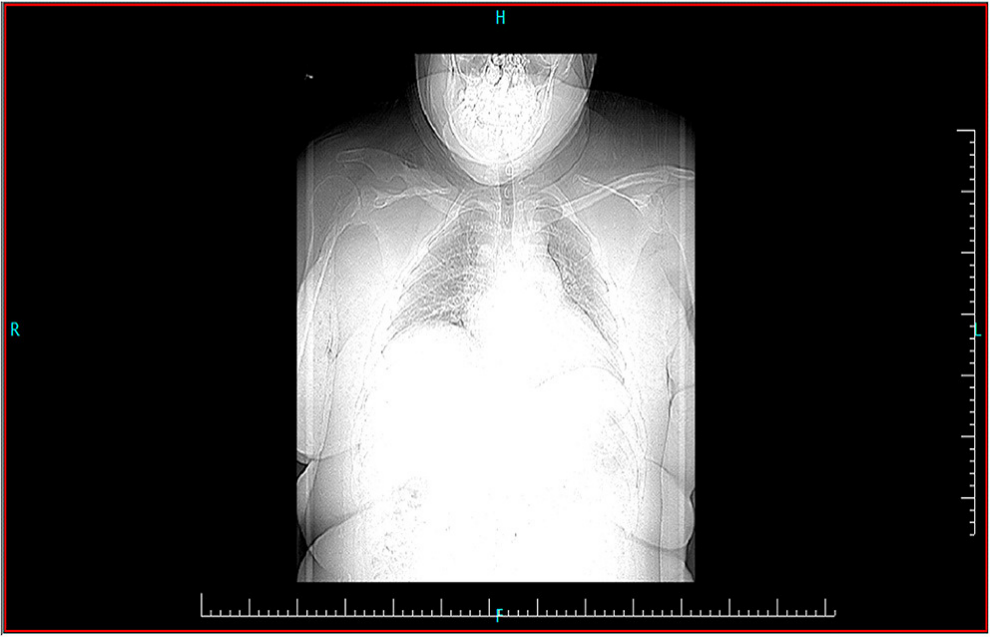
Preoperative computed tomography scan revealing the scope of the lesion. This was essential in identifying the small bone fragments separated from the fracture.
